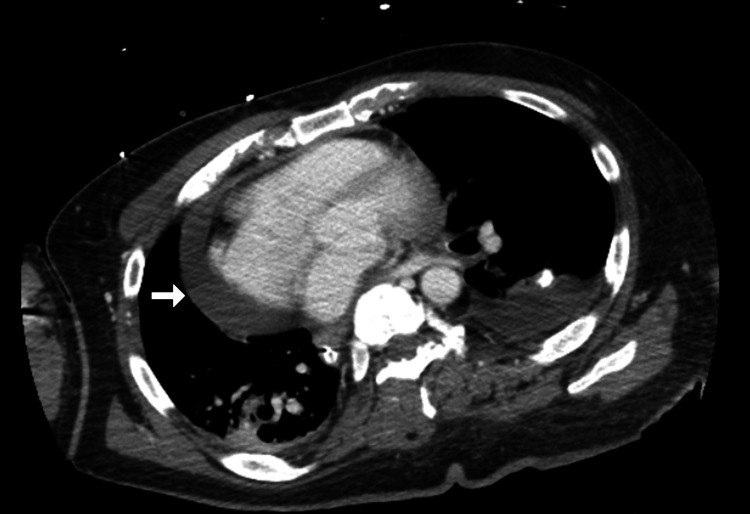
CT scan of the chest after the procedure
